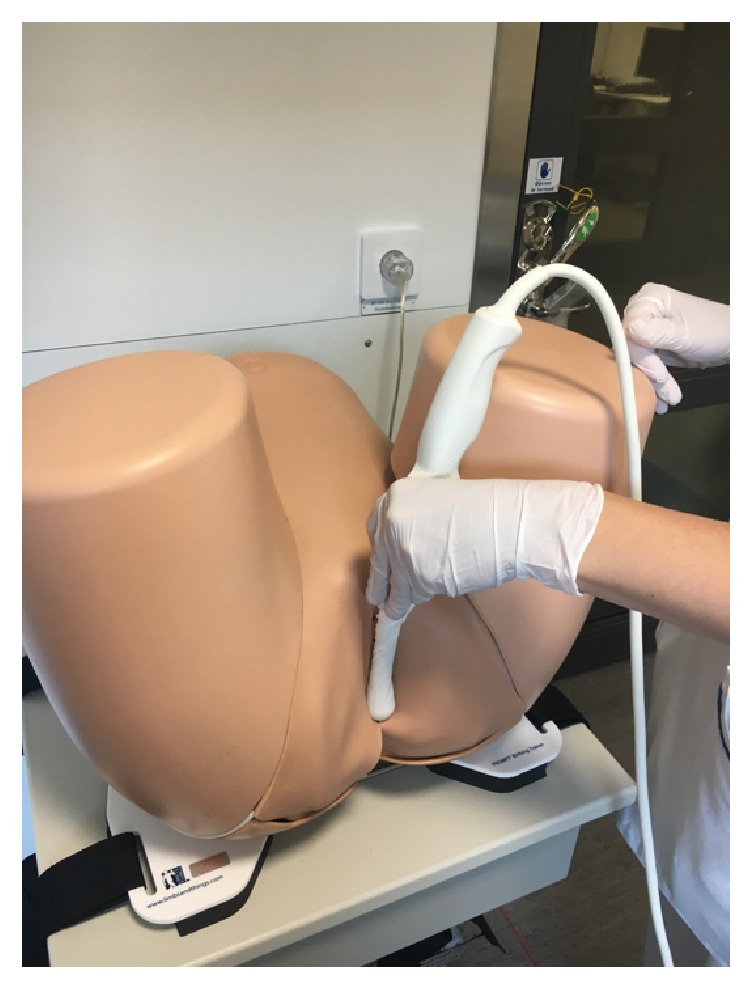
Transperineal ultrasound method to determine the anovaginal distance.
